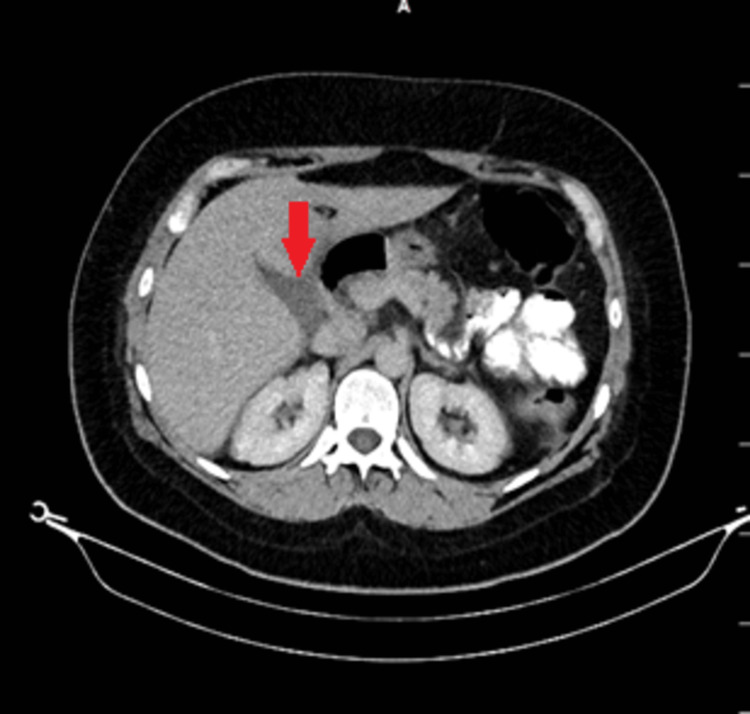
Abdominal CT showed (red arrow) fluid collection on the gallbladder bed. CT: computed tomography
